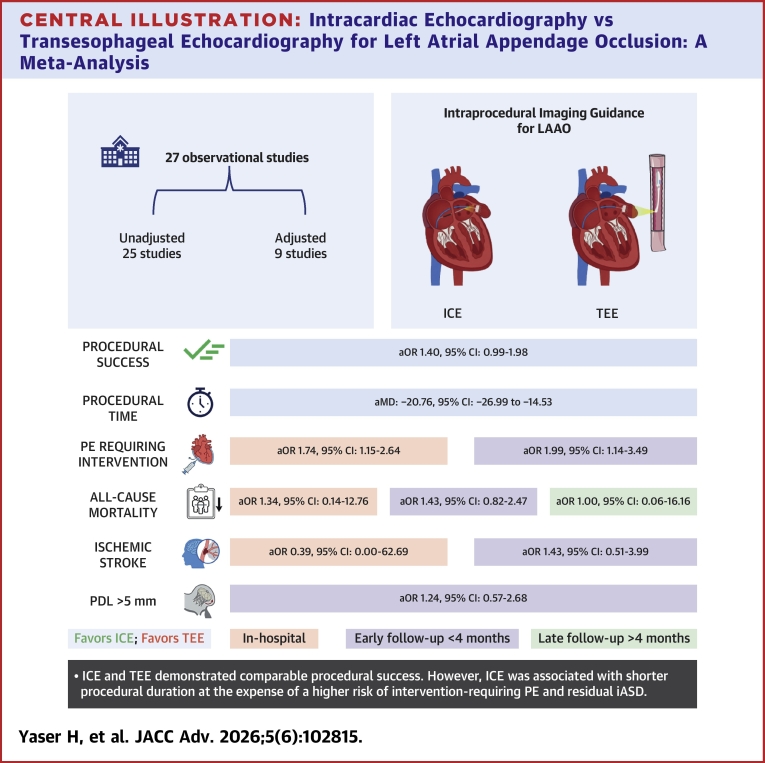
Intracardiac Echocardiography vs Transesophageal Echocardiography for Left Atrial Appendage Occlusion: A Meta-Analysis In this meta-analysis of 27 observational studies, intracardiac echocardiography and transesophageal echocardiography demonstrated comparable procedural success for intraprocedural guidance of left atrial appendage occlusion. However, intracardiac echocardiography was associated with shorter procedural duration at the expense of a higher risk of intervention-requiring pericardial effusion and residual iatrogenic atrial septal defect. aMD = adjusted mean difference; aOR = adjusted OR; iASD = iatrogenic atrial septal defect; LAAO = left atrial appendage occlusion; PDL = peridevice leak; PE = pericardial effusion; TEE = transesophageal echocardiography; other abbreviations as in Figures 1 and 2.
